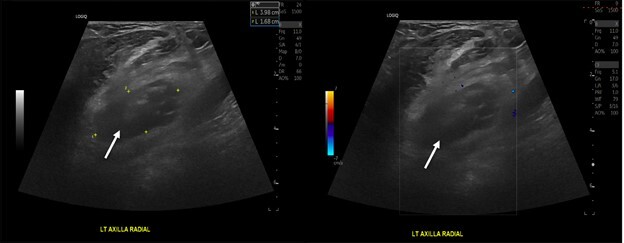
(Left panel) White arrow indicates point-of-care ultrasound (POCUS) image of abnormal left axillary lymph node on B-mode. (Right panel) White arrow points to POCUS image of abnormal left axillary lymph node with no vascularity on color Doppler mode.
